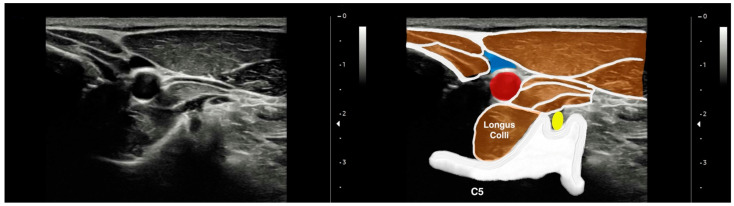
Ultrasound imaging of the longus colli muscle at the C5 level. Yellow: C4 root; blue: interior jugular vein; red: common carotid artery.
